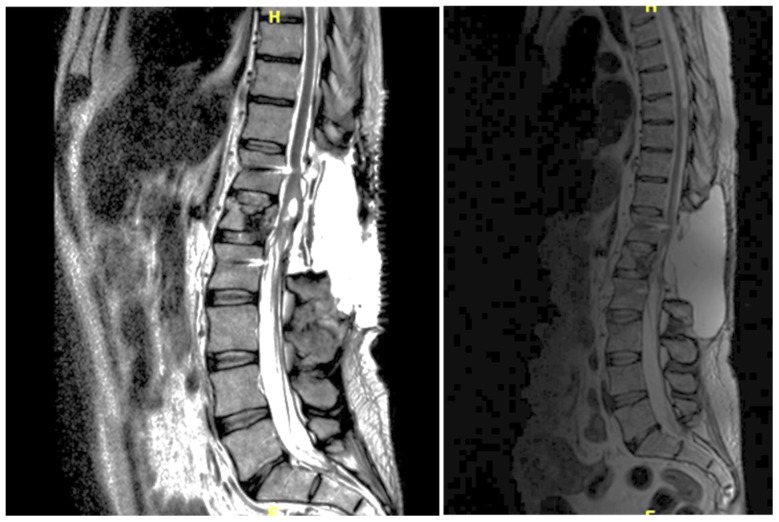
Case 1: pre-operative MRI (left) and post-operative MRI (right).
